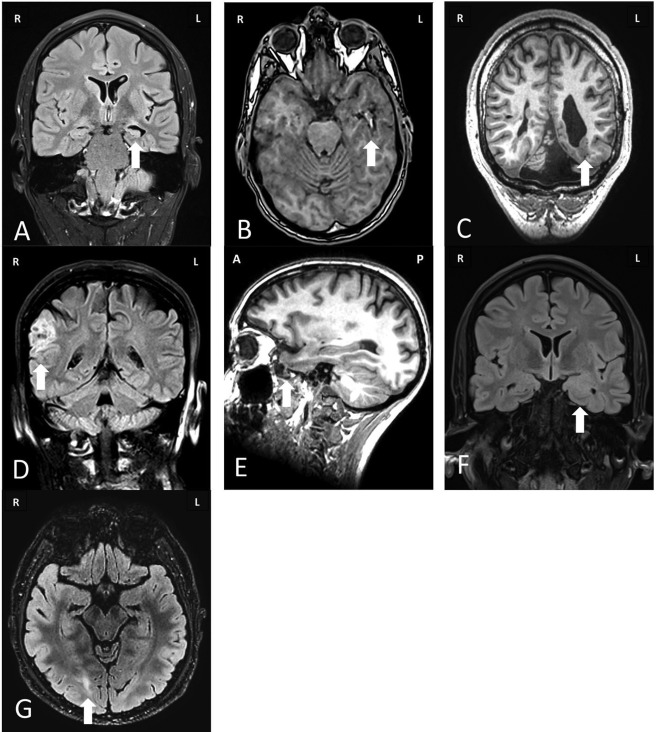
Magnetic resonance imaging of common pathologies underlying drug‐resistant focal epilepsy that are amenable to surgical treatment. (A) Coronal fluid‐attenuated inversion recovery (FLAIR) image showing increased T2 signal in the left hippocampus associated with volume loss and compensatory dilatation of the left temporal horn consistent with left hippocampal sclerosis. (B) Nonenhanced axial T1‐weighted image of a patient with a lesion in the left temporal lobe that has a “popcorn” appearance due to a hemosiderin ring and mixed intensity blood products consistent with a cavernoma. (C) Nonenhanced coronal T1‐weighted image of a patient with multiple bilateral well‐demarcated periventricular lesions that have imaging characteristics matching gray matter consistent with nodular periventricular heterotopia. This is associated with polymicrogyrialike overlying cortex. Note is also made of a posterior fossa arachnoid cyst and ventricular asymmetry. (D) Coronal FLAIR image of a patient with a sharply demarcated cortically based “pseudocystic” lesion in the right supramarginal gyrus that returns a hyperintense signal, consistent with a dysembryoplastic neuroepithelial tumor. There is associated overlying calvarial remodeling. (E) Sagittal T1‐weighted image through the left temporal lobe revealing herniation of the temporal pole through the floor of the middle cranial fossa consistent with a meningoencephalocele. (F) Coronal FLAIR image with increased signal and expansion of the left amygdala. Contrast‐enhanced imaging did not reveal any enhancement, consistent with a diffusely infiltrating low‐grade glioma. (G) Axial FLAIR image revealing increased signal in the right occipital lobe with blurring of the cortical–subcortical margin consistent with type 2B focal cortical dysplasia.
